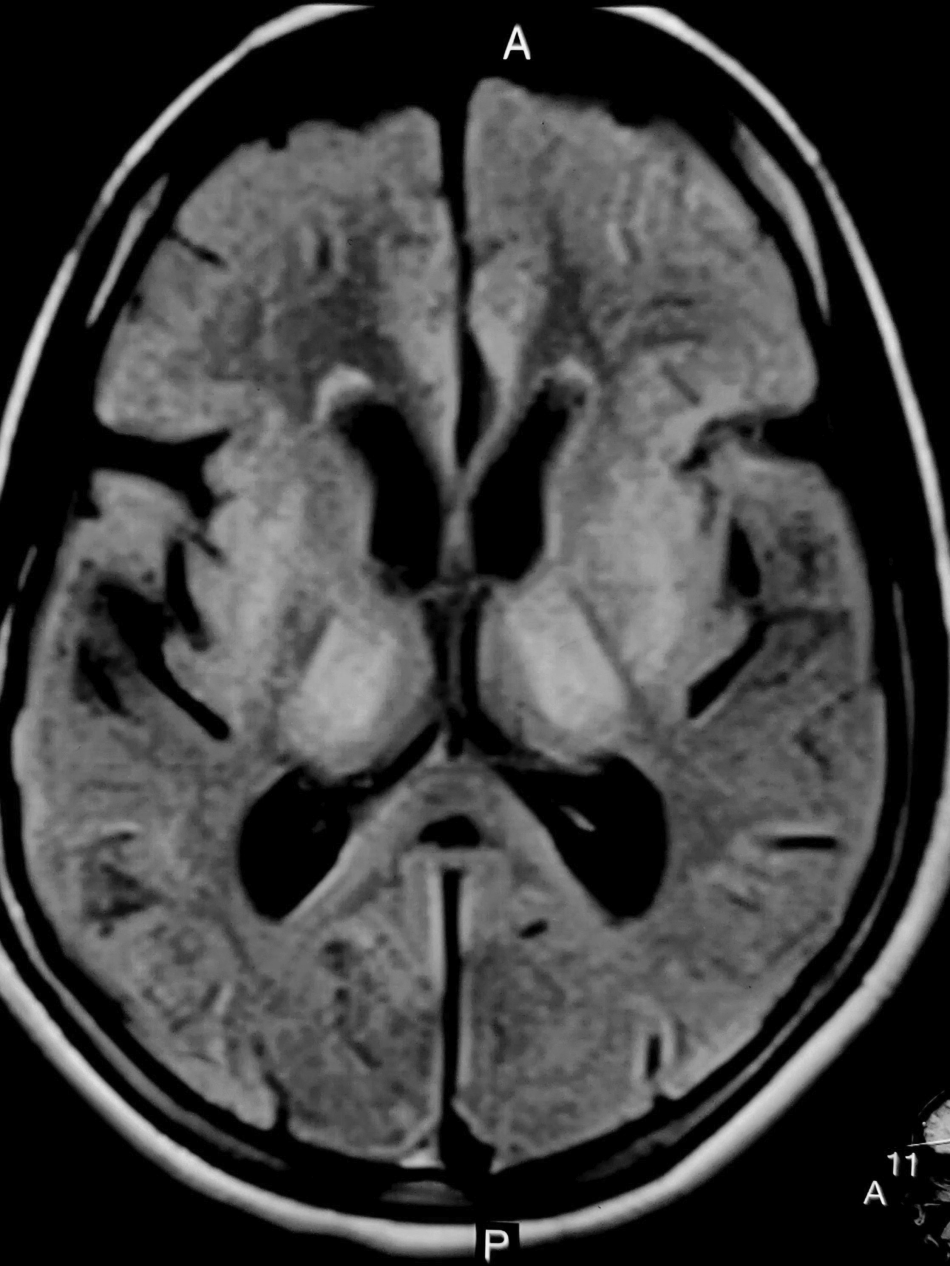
FLAIR hyperintensity in the putamen and thalamus.
